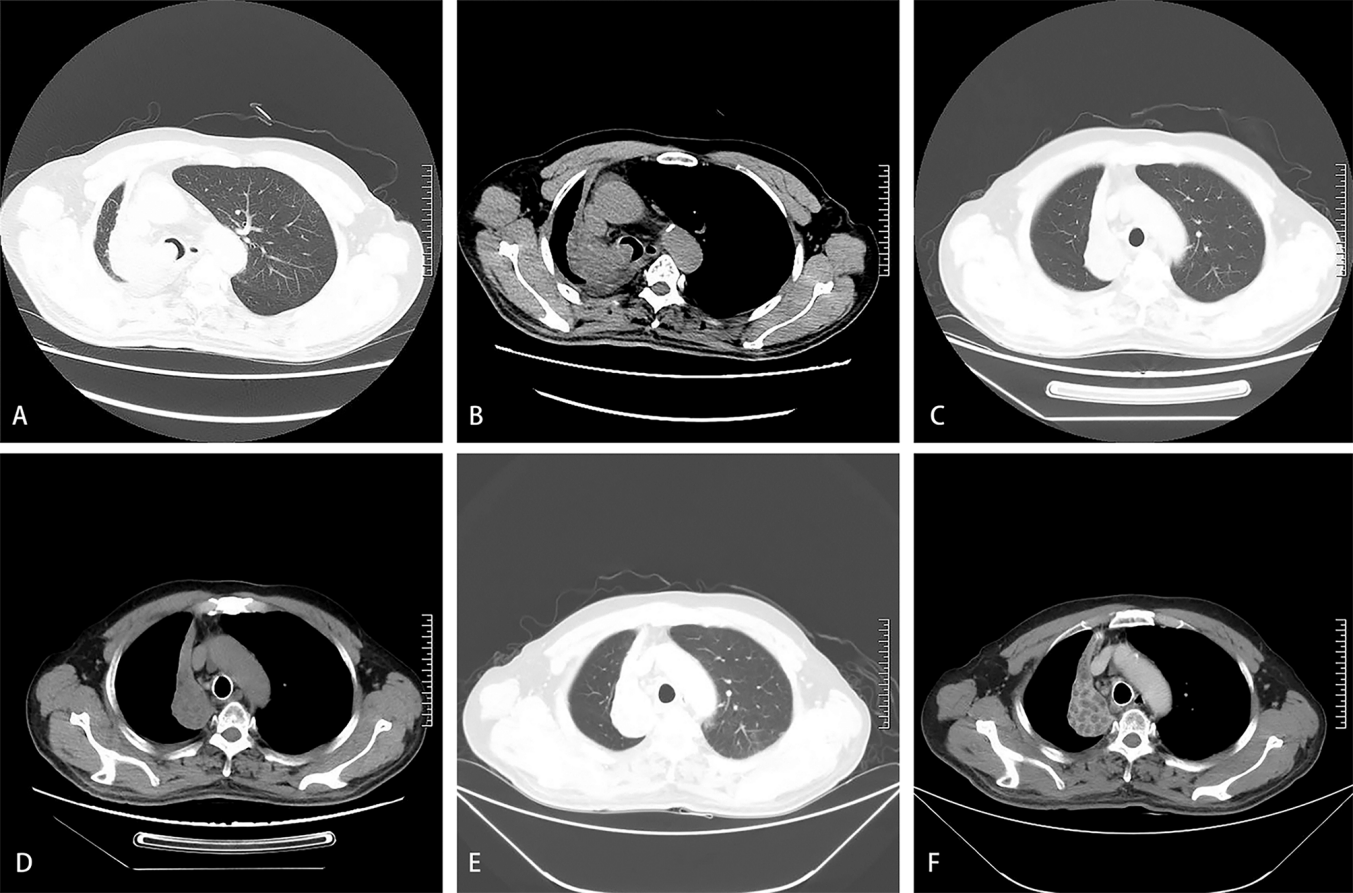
A 61-year-old man was diagnosed with squamous cell carcinoma of the right lung 8 months ago. He had progressive dyspnea for 3 days. The dyspnea score was 5. Chest computed tomography on admission showed complete atelectasis in the lung window (A) and the mediastinal window (B). Repeated computed tomography in the lung window (C) and the mediastinal window (D) showed a reduction in the size of the tumor in the right lung one month after the first BA-TACE. Repeated computed tomography in the lung window (E) and the mediastinal window (F) showed obvious necrosis in the tumor area one month after the second BA-TACE.
